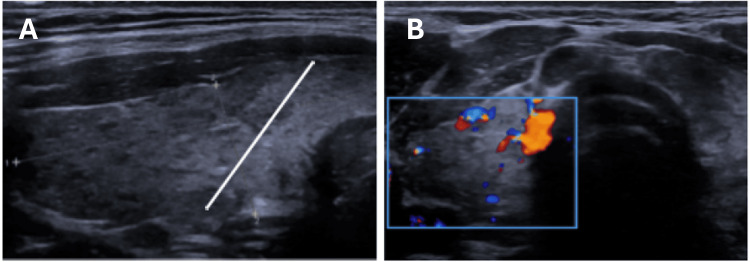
Thyroid ultrasonography Thyroid ultrasonography shows diffusely hypoechoic and heterogeneous echotexture (mainly to the left of the white line, as shown in Image A), with increased vascularization (blue rectangular shape, as shown in Image B).
